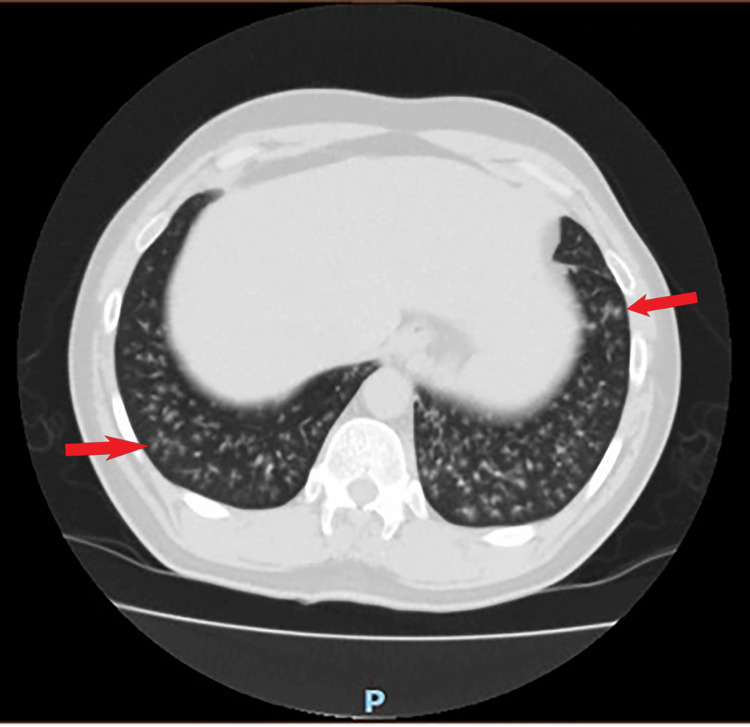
The HRCT shows multiple miliary small nodules in both lung bases. HRCT: high-resolution computed tomography
